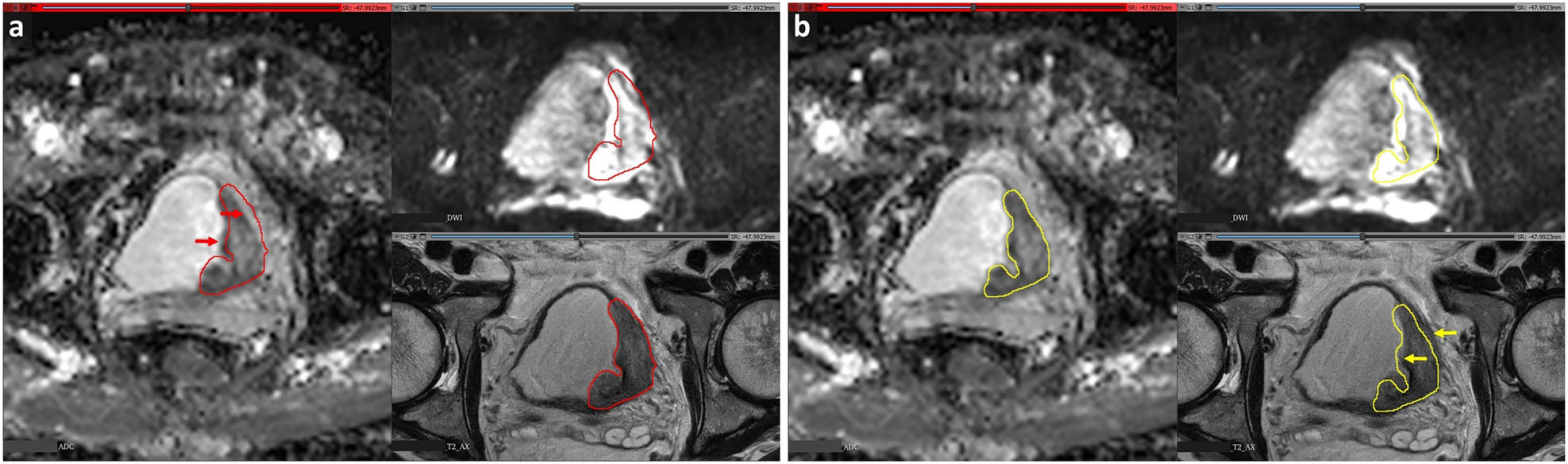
Axial MRI images using a 3D Slicer interface designed for segmentations of bladder cancer that allows for the simultaneous display of multiple sequences (ADC, DWI, and T2W). Comparison is presented between a segmentation performed on T2W images (a) vs one performed on ADC images (b). Notice the differences in border alignment and shape as these segmentations are being transposed on the other sequences
